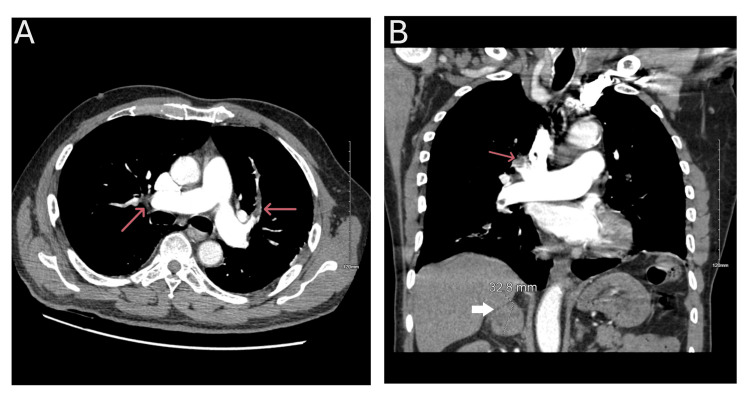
Computed tomography (CT) angiogram of the chest showing extensive bilateral pulmonary emboli with central pulmonary artery enlargement. A. Transverse section showing embolus in the left and right pulmonary arteries (red arrows).
